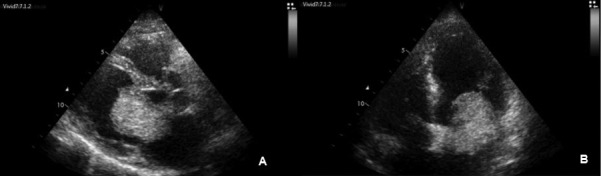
Echocardiography showing giant left atrial myxoma on parasternal long axis (A) and apical 4 chamber (B) imaging.
